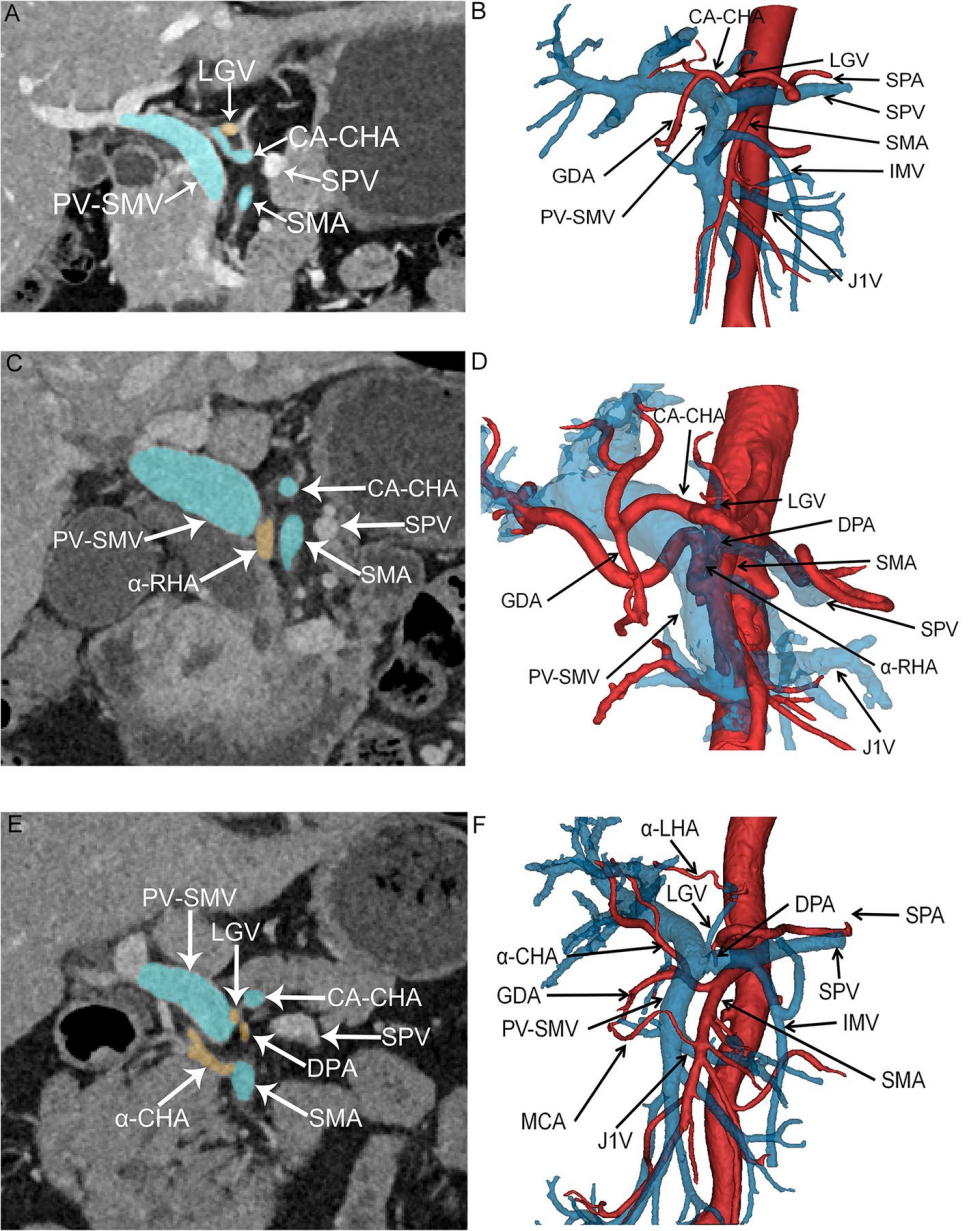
Coronal CT images and three-dimensional reconstructed images of the type II Heidelberg triangle. A, C, E Coronal CT images depicting the type II Heidelberg triangle, with named vessels crossing the interior of the Heidelberg triangle, accounting for 46.2% of reconstructed patients. The blue area represents the boundary vessels of the Heidelberg triangle, and the orange area indicates the vessels crossing through the interior of the Heidelberg triangle. The white arrowhead points to vessels around the Heidelberg triangle. B, D, F The three-dimensional reconstructed image of the type II Heidelberg triangle. The black arrowhead points to vessels around the Heidelberg triangle. Veins are depicted in blue, while arteries are represented in red. Abbreviations: CA, celiac axis; CHA, common hepatic artery; SMA, superior mesenteric artery; DPA, dorsal pancreatic artery; SPA, splenic artery; LGA, left gastric artery; GDA, gastroduodenal artery; IPDA, inferior pancreaticoduodenal artery; α-RHA, variant right hepatic artery; α-LHA, variant left hepatic artery; α-CHA, variant common hepatic artery; MCA, middle colic artery; J1A, first jejunal artery; LGV, left gastric vein; SMV, superior mesenteric vein; PV, portal vein; SPV, splenic vein; IMV, inferior mesenteric vein; J1V, first jejunal vein
